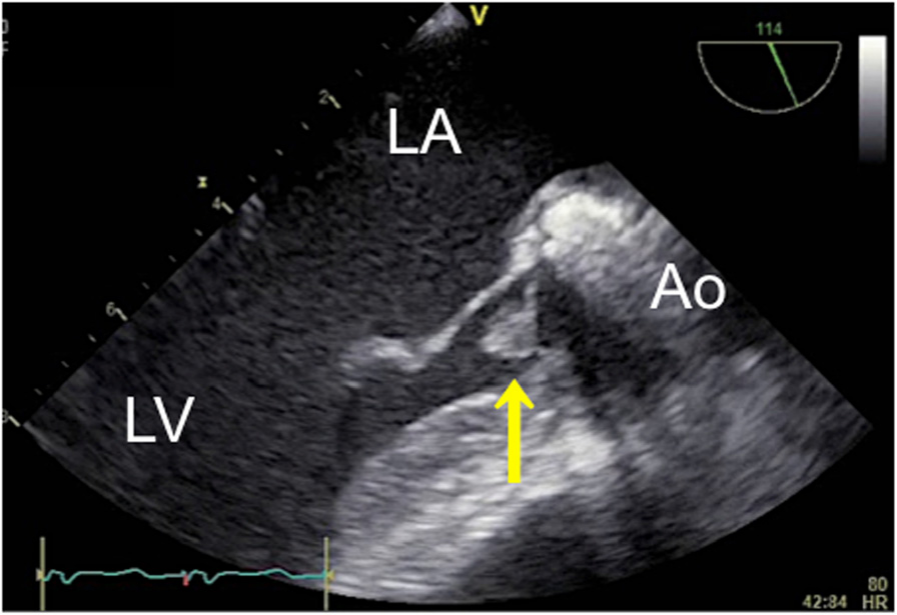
Two-dimensional transesophageal echocardiography image. This long-axis view of the aortic valve shows a mobile membranous structure attached to the aortic valve (yellow arrow). Ao, aorta; LA, left atrium; LV, left ventricle.
